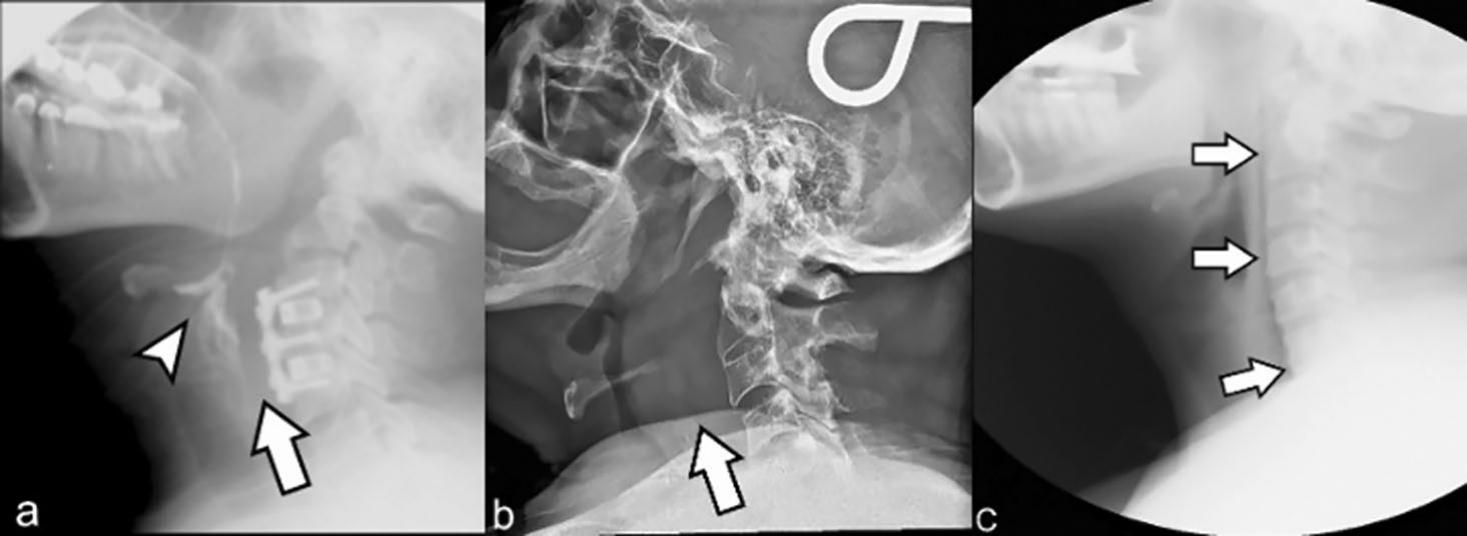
Abnormal prevertebral soft tissues on MBSS in 3 separate patients. A First patient, 1-day post-anterior cervical discectomy and fusion (ACDF) complaining of dysphagia. Prevertebral soft tissue thickening is present anterior to the fusion hardware (arrow). Laryngeal flash penetration is subtly present as well (arrowhead). On follow-up MBSS performed 6 days later (not shown), the soft tissue thickening had significantly decreased and no laryngeal penetration or aspiration was demonstrated. In the absence of an underlying fluid collection, prevertebral soft tissue swelling after ACDF will usually peak around 2–4 days and then gradually improves/decreases, resolves within 6 weeks. B Second patient complaining of neck pain and dysphagia, without recent surgical or trauma history. Prevertebral soft tissue thickening is present (arrow). Further clinical and imaging evaluation was recommended, which revealed discitis osteomyelitis due to methicillin-susceptible Staphylococcus aureus. C Third patient with history of asthma complaining of chest and neck pain, as well as dysphagia. Subtle linear lucency tracking along the prevertebral soft tissues (arrows), consistent with retropharyngeal emphysema. Chest radiograph demonstrated pneumomediastinum (not shown). Acknowledgement: Cases 2 and 3 are courtesy of Dr. Sarah Shaves, Eastern Virginia Medical School
